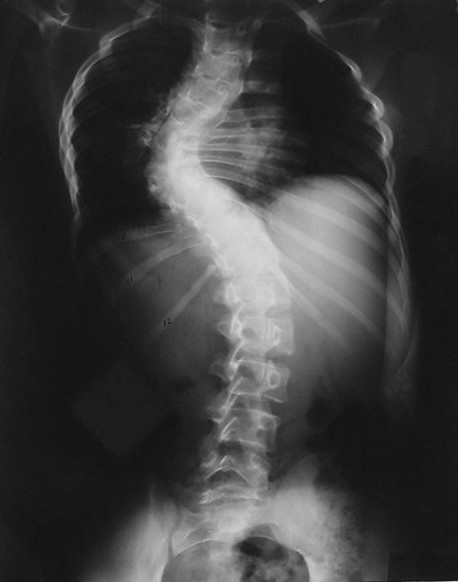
Preoperative standing posteroanterior radiograph showing left dystrophic scoliosis measuring 75 degrees from T4 to the T10.
